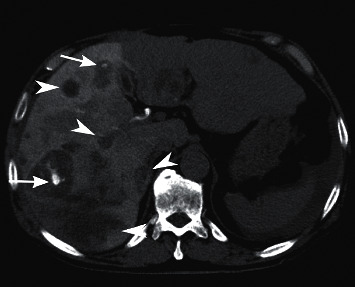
Arterial phase of computed tomography during right hepatic arteriography. Pooling of contrast medium was observed (arrows). Contrast effect of tumors was low (arrowheads) and it was considered to indicate decrease in vascularity of hepatocellular carcinomas.
